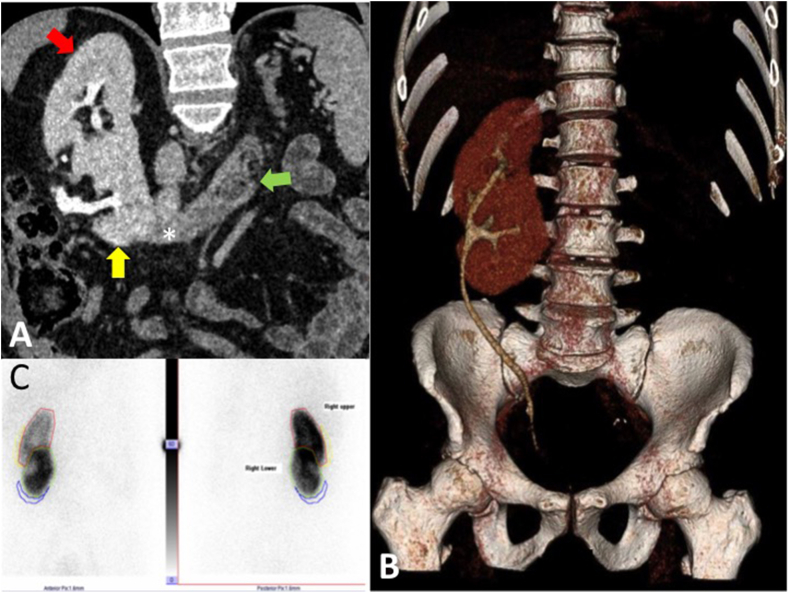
(A) Coronal oblique contrast enhanced CT reconstruction in the excretory phase shows two kidneys in the right lumbar region. The upper kidney (red arrow) has an anterior orientation whilst the lower partially fused kidney (yellow arrow) has an anterolateral orientation. The atrophic left kidney with poor cortical enhancement and no contrast excretion is seen on the left (green arrow) and is joined to the supernumerary right kidney by a fibrous parenchymal bridge (asterisk). (B) 3 D reconstruction of contrast enhanced CT excretory phase showing right supernumerary kidney with lower kidney renal pelvis rotated anterolateral and both ureters joining together and taking a normal course to insert into the urinary bladder. The atrophic left horseshoe kidney shows poor parenchymal enhancement and no contrast excretion and therefore is not seen on this image. (C) DMSA scan shows all the activity in the right supernumerary kidney with no activity in the atrophic horseshoe left kidney. (For interpretation of the references to colour in this figure legend, the reader is referred to the Web version of this article.)
